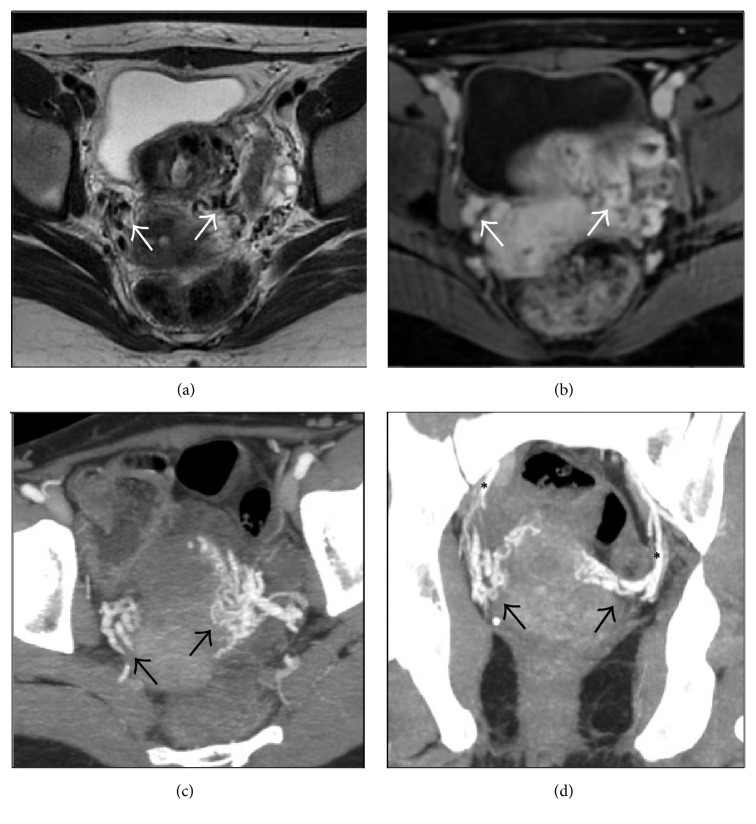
MR ((a) and (b)) and CT ((c) and (d)) before the embolization. (a) T2-weighted MR in axial plane; (b) contrast-enhanced T1-weighted MR in axial plane; arterious contrast-enhanced CT with maximum intensity projection in axial (c) and coronal planes (d). Multiple ectatic and serpiginous anomalous vessels adjacent to the uterine wall bilaterally are appreciable at MR and CT imaging; these are indicated by white arrows in (a) and (b) and black arrows in (c) and (d). In (d), uterine arteries, indicated by black asterisks, are in direct connection with the anomalous vessels of the uterine wall.
